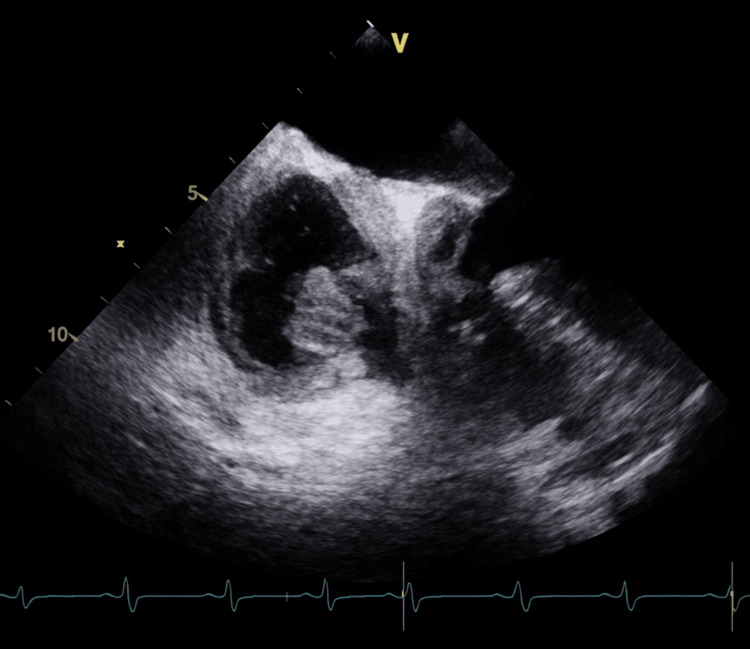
Echocardiography showing right atrial mass
